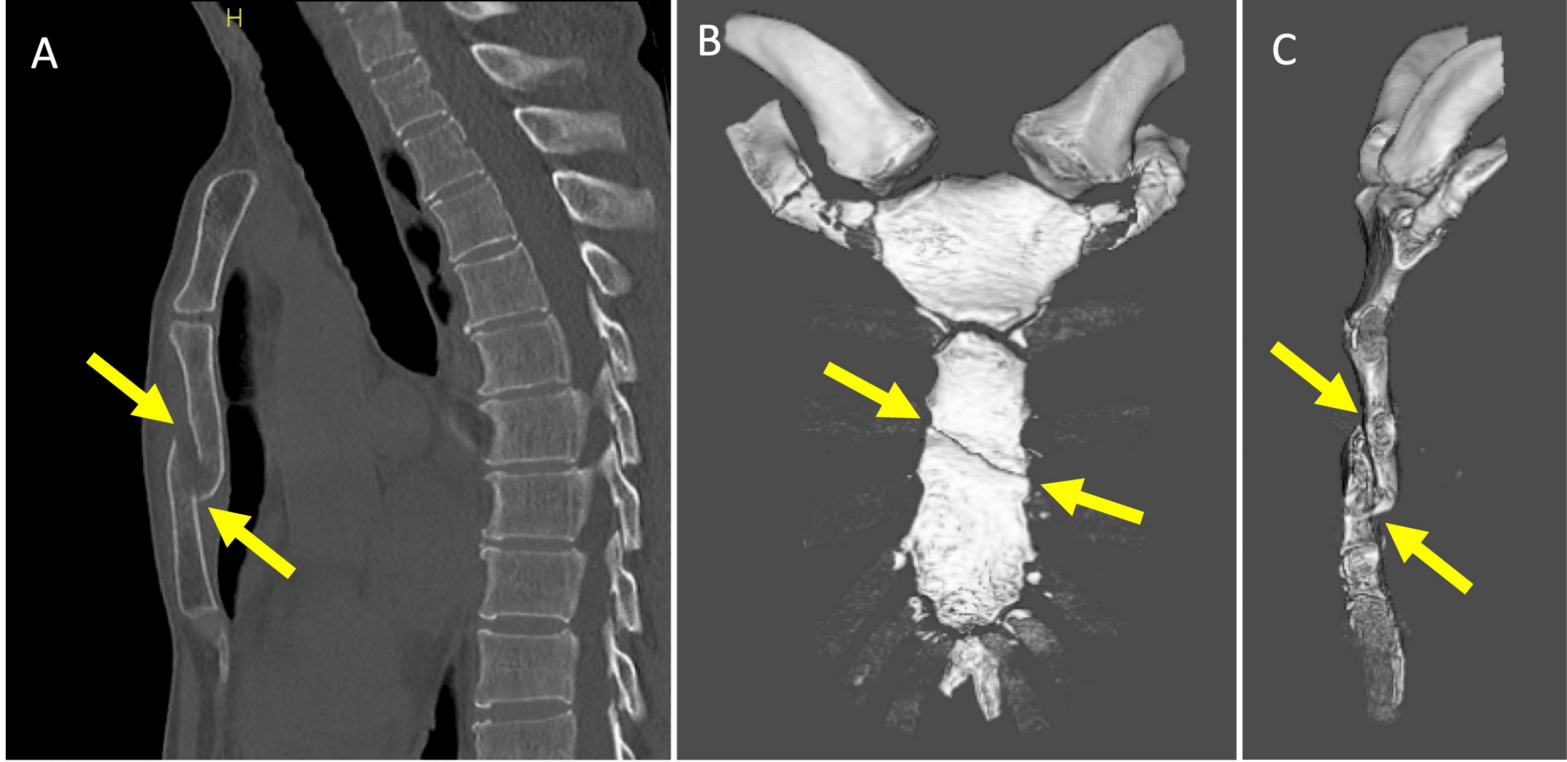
Preoperative imaging of sternal fracture (A) Sagittal CT image showing fracture site (arrow); (B) Anterior view of three-dimensional reconstructed CT image with fracture line (arrow); (C) Lateral view of three-dimensional reconstructed CT image with fracture location (arrow) CT, Computed tomography
